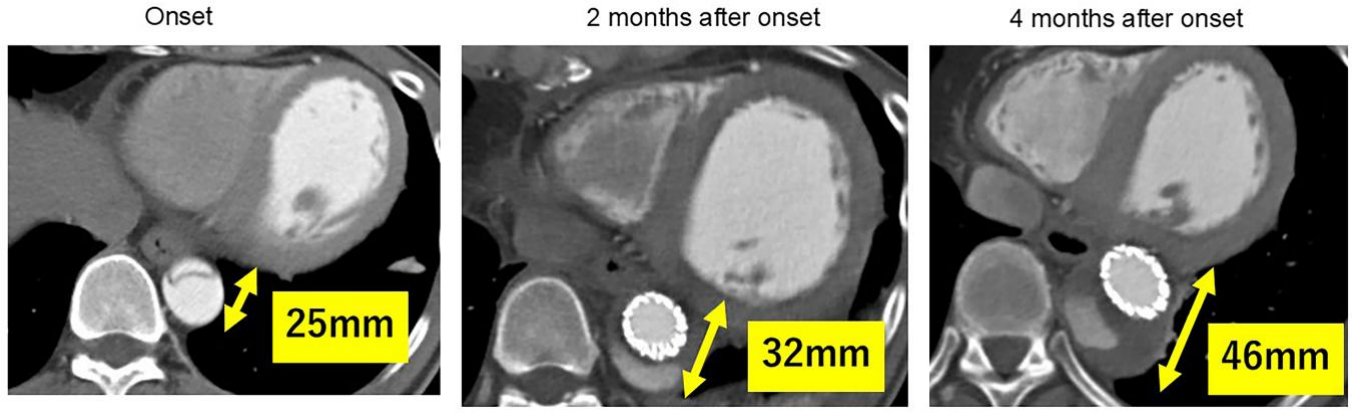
Time-course data of the aortic diameter showing progressive enlargement on serial contrast-enhanced computed tomography. The maximum diameter increased from 25 mm at onset to 32 and 46 mm after 2 and 4 months after onset, respectively, prompting surgical intervention.
